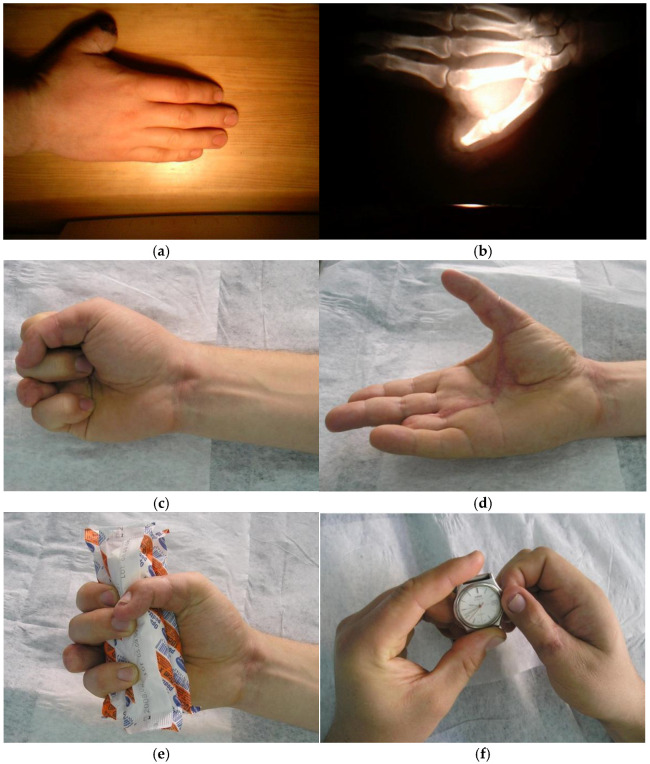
A case of a 25-year-old male manual worker who was admitted for thumb function reconstruction after amputation and treatment at another centre. The patient was offered pollicisation of the distal part of the fourth finger at the level of the proximal interphalangeal joint. (a) Initial presentation of the thumb, three months after amputation; (b) X-ray of the hand on arrival at our centre; (c,d) Final presentation showing a satisfactory aesthetic outcome after the procedure, with the thumb flexed and abducted; (e,f) Functional result showing good grasp and key pinch of the operated limb six months after the described surgical treatment.
